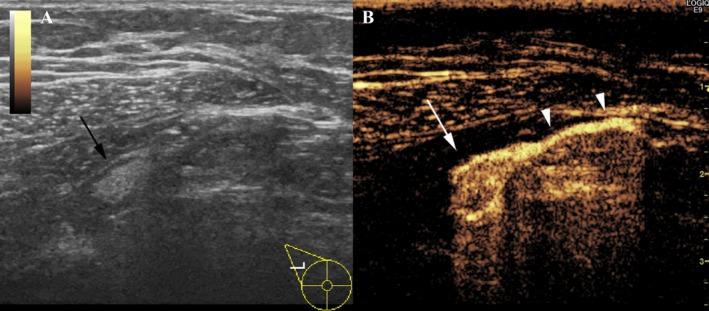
Representative results of dual monitoring of right axillary lymph node basin by Gray‐scale and contrast‐enhanced ultrasound of a patient who received periareolar injection of Sonazoid. (A) Gray‐scale ultrasound image shows elliptical lymph node with fatty hilum (black arrow). (B) Contrast‐enhanced ultrasound image clearly shows that contrast‐enhanced sentinel lymph node (white arrow) is connected to contrast‐enhanced lymphatic vessel (white arrow heads).
